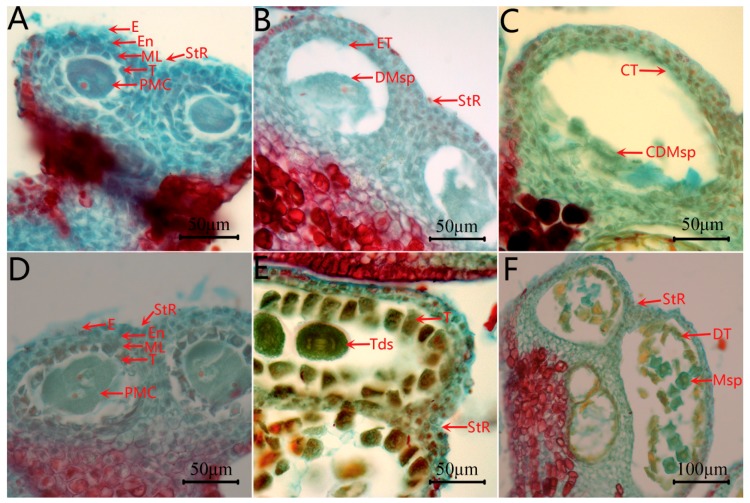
Phenotypic comparison between C2P5A and C2P5B at different development stages. (A–C) CMS line C2P5A; (D–F) maintainer line C2P5B. (A,D) Pollen mother cell stage (Pms); (B,E) Tetrad stage (Tds); (C,F) Mononuclear stage (Ms). PMC, pollen mother cell; E, epidermis; En, endothecium; ML, middle layer; Msp, microspores; T, tapetum; DT, degenerated tapetum; Tds, tetrads; Mn, mononuclear; DMsp, degenerated microspores; CDMsp, complete degenerated microspores; CT, compact tapetum; ET, entire tapetum; StR, stomium region.
